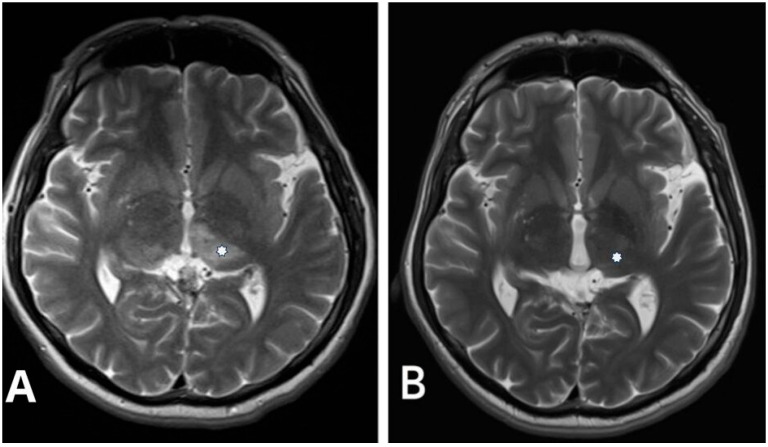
(A) Pre-procedural T2-weighted axial image in the same patient with Cognard type IV falcotentorial DAVF showing a bilateral thalamic edema (asterisk). (B) Control postprocedural T2-weighted axial image performed 2 years after intervention demonstrates complete resolution of the thalamic edema (asterisk).
